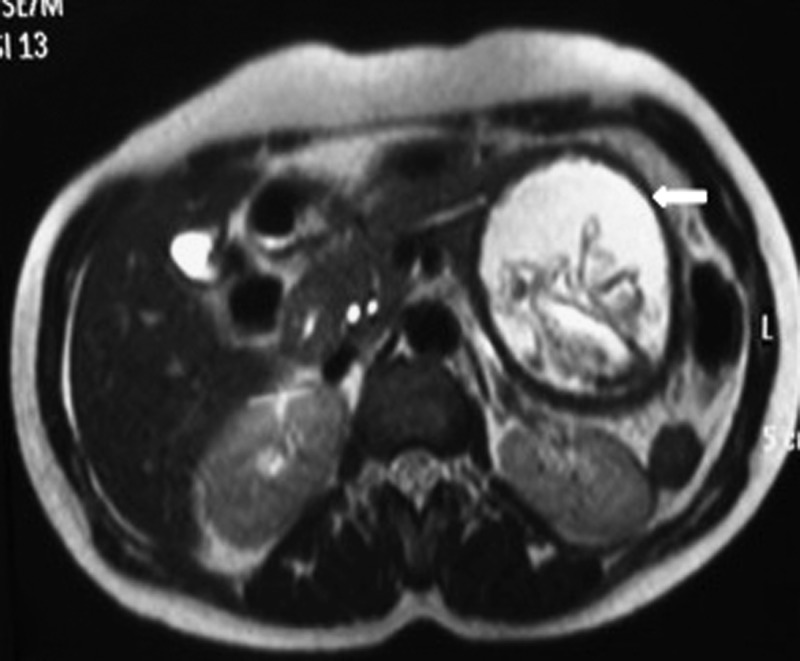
Magnetic resonance imaging abdomen axial T2-weighted image showing a well-defined altered signal intensity lesion with a thick complete hypointense rim and detached membranes from the distal body and tail of the pancreas (bold white arrow).
